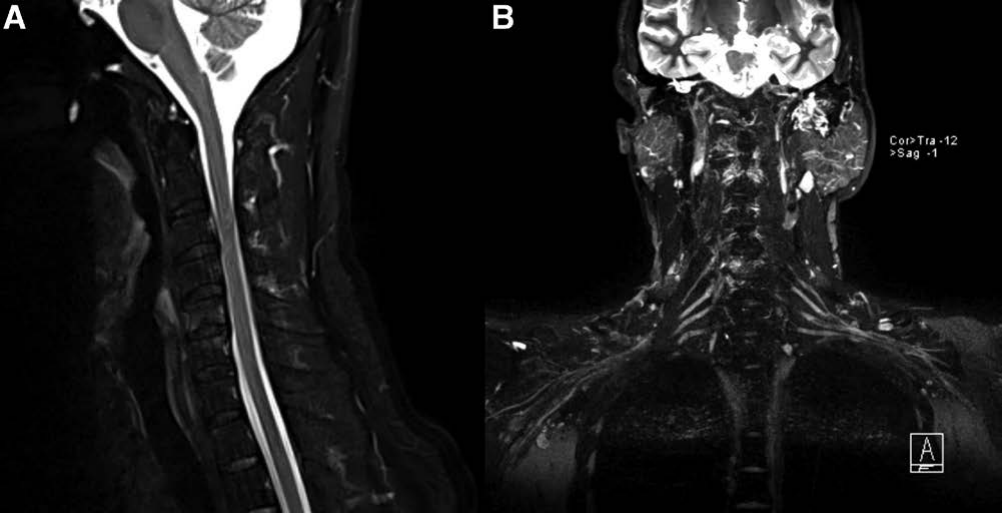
(A) Cervical spine MRI results: Cervical degenerative changes (C2/3–C6/7 intervertebral disc degeneration, mildly bulged C3/4–C6/7 intervertebral discs, C4–7 vertebral hyperostosis, and endplate inflammation between C5 and C6 vertebrae), (B) Bilateral brachial plexus MRI results: No evident bilateral brachial plexus injury.
